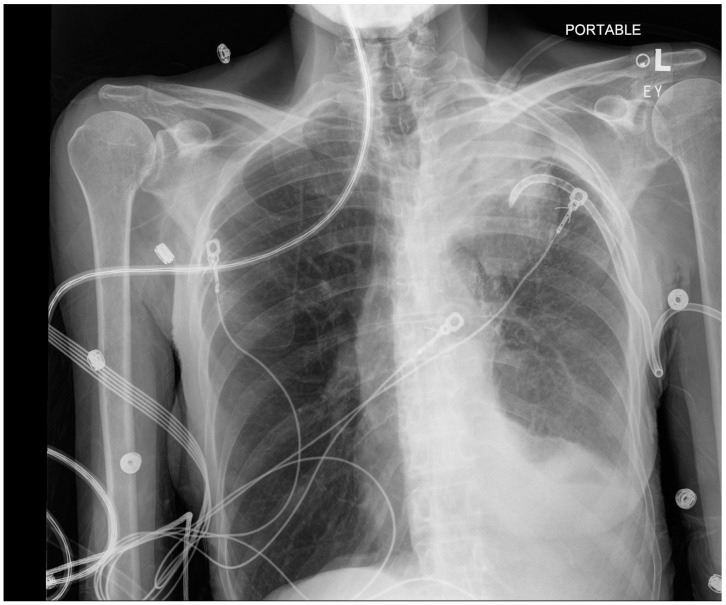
CXR left upper lobe volume loss (day 2). Moderate left pleural effusion compatible with hemothorax (which was seen on CT scan). Stable cardiac silhouette. Left apical chest tube. Left hilar endobronchial valves. L—indicates left side, EY—the initials of the radiographer.
